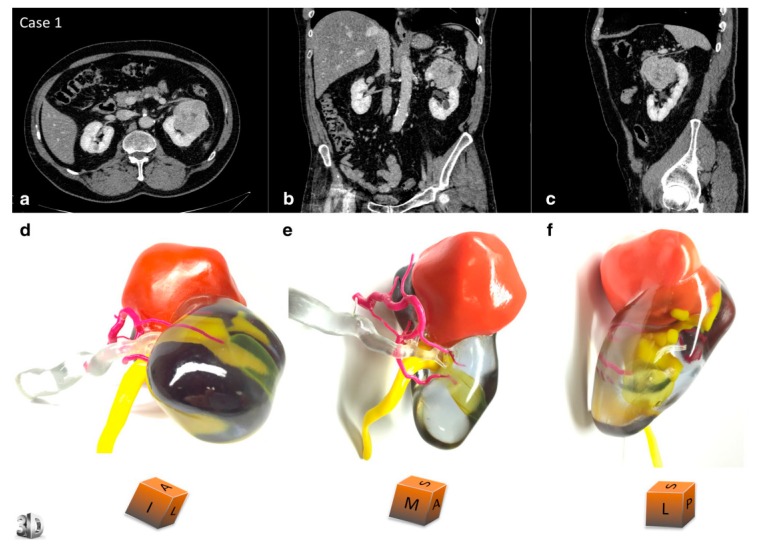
3D-printed model of a 67-year-old male with renal tumour at the upper pole of left kidney. Comparative views of the CT scan at the nephrographic phase ((a) axial, (b) coronal, and (c) sagittal planes) and corresponding views of the physical model ((d) superior and median view, (e) median and anterior view, and (f) lateral view). An inferior polar cyst is also displayed on this model (translucent yellow). The cubes show the 3D-printed model orientation in space (I = inferior face, A = anterior face, L = lateral side, S = superior face, P = posterior face, M = median side). The patient underwent a left radical nephrectomy for a 65 × 56 × 42 mm clear cell renal cell carcinoma, pT1bN0Mx, Fuhrman grade 3. The arterial tree is presented in opaque magenta, the collecting system in opaque yellow, and opaque orange for tumour display. The renal vein and renal parenchyma are kept translucent to allow the best visualisation of the relationships between the renal tumour and surrounding structures. Reprinted with permission from Bernhard et al. [6].
